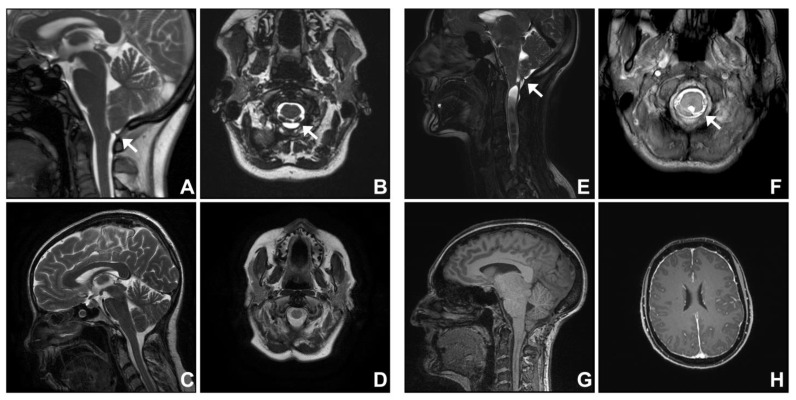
Chiari mimic: dural band. A 41-year-old woman (A–D) presented with a 4-year history of occipital headache and neck and bilateral upper limb pain. Cardiac gated steady-state free precession (SSFP or bFFE) imaging as well as thin-slice, heavily T2-weighted images showed cerebellar tonsillar descent, a thick dural band compressing the cerebellar tonsils ((A,B); white arrows), and a resultant reduction of cisterna magna (A,B). Decompression surgery and division of the dural band restored the cisterna magna to a normal size (C,D). A 49-year-old woman (E–H) presented with a 9-year history of hearing changes and sensory disturbance in the left upper limb and trunk. An MRI showed cerebellar tonsillar descent 7 mm below the foramen magnum up to the C1 arch with a communicating cervico-thoracic syrinx (E). In addition, compression of the tonsils at the cervico-medullary junction was evident from a distinct septation posteroinferior to the cerebellar tonsils ((E,F); white arrows) on cardiac-gated cine bFFE sequence (E,F). Following posterior fossa decompression, there was persistent cerebellar tonsillar descent, and imaging features were indicative of intracranial hypotension: prominent dural venous sinuses, diffuse pachymeningeal enhancement, pituitary gland enlargement, flattening of the pons, and dorsal beaking of the tectum (G,H).
